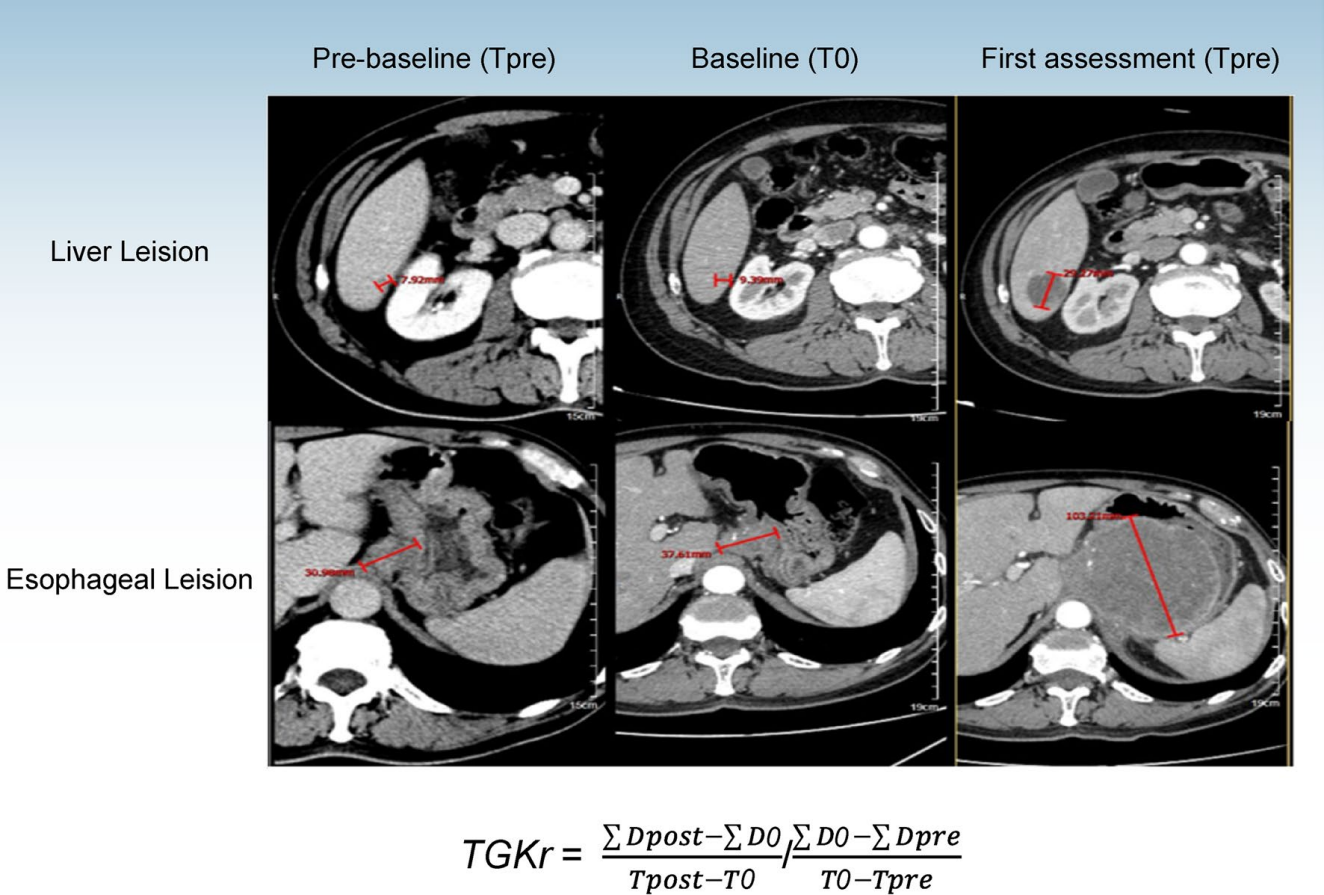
Thoracic and abdominal CT review results showed accelerated tumor progression. A. Pre-baseline assessment: the cross-sectional diameter of the right lobe of the liver was 4.62 mm, and the cross-section diameter of the lesion with a slight density of the esophageal was 21.17 mm; B. Baseline assessment: the cross-sectional diameter of the right lobe of the liver was 8.73 mm, and the cross-section diameter of the lesion with a slight density of the esophageal space was 28.17 mm; C. First assessment after ICI evaluation: the cross-sectional diameter of the right lobe of the liver was 42.18 mm, and the cross-section diameter of the lesion with a slight density of the esophageal space was 101.02 mm
